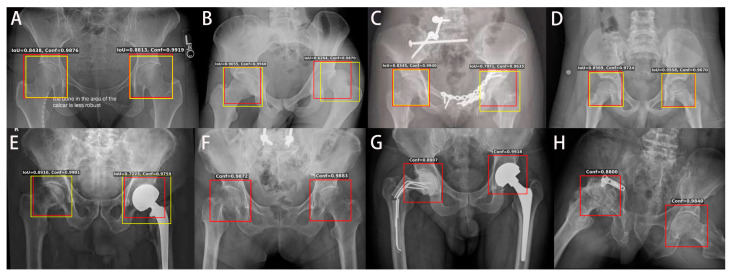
Visualization of hip ROI detection results on the testing dataset. Yellow boxes indicate manual labels, and red boxes indicate detected hip ROIs. In all scenarios, the ROI could be detected well in both hips. (A) A radiograph with some text outside the key hip area. (B) A radiograph with left hip dislocation. (C) A radiograph with plates on the left pubic ramus and acetabulum. The hips were detected correctly. (D) A radiograph of pediatric patients. (E) A radiograph showing left hip replacement and deformity of the right hip. (F) Right proximal femoral fracture. (G) The right hip showing a fracture of a temporal cemented prosthesis and left total hip replacement. (H) Right acetabular fixation with plate with destructed femoral head.
